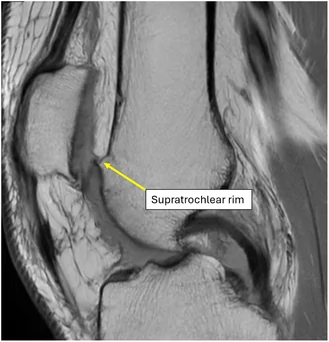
A sagittal MRI slice of the left knee joint in a patient with anterior knee pain, showing the medial supratrochlear rim. The rim is indicated by a yellow arrow. The depicted cross‐section is the plane where the anterior outline of the medial femoral condyle first becomes visible, along with the supratrochlear rim. MRI of the knee is necessary to confirm the presence of the rim before planning surgery and to assess any patellar articular cartilage damage. (MRI, magnetic resonance imaging.)
